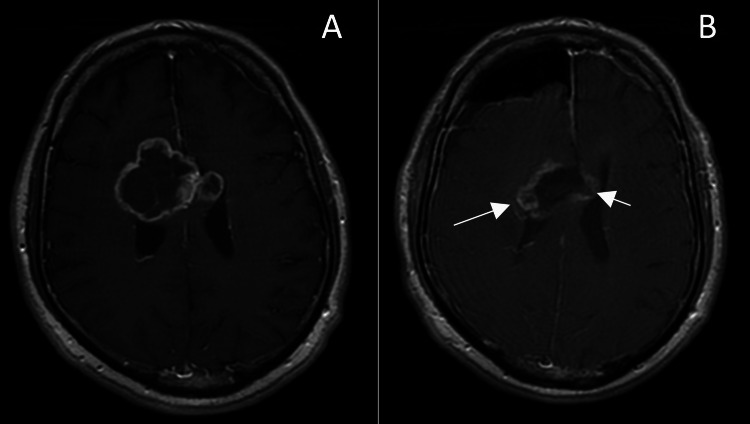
Follow-up MRIs of patient no. 3. (A) Preoperative T1-enhanced MRI image; (B) Day 1 postoperative image with residual tumor volume of 10.27 cm3 (arrows).
